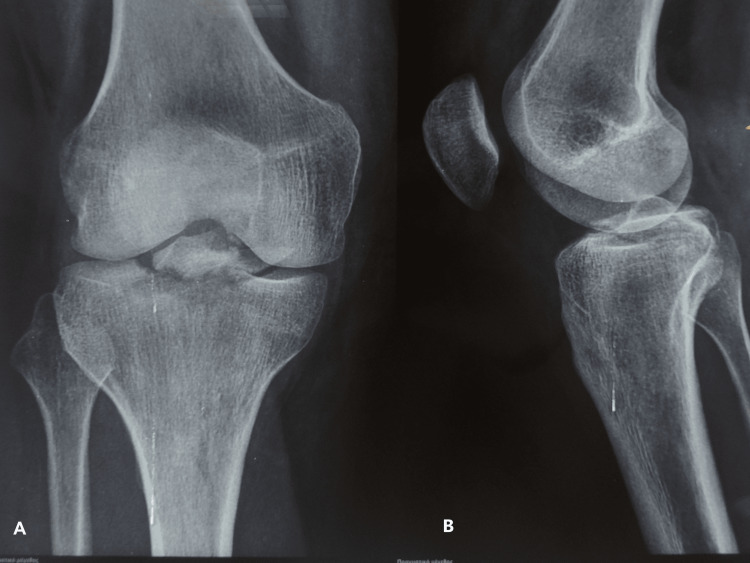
Right knee X-ray. (A) Anteroposterior view. (B) Lateral view. Patient's radiographs depicting the ACL-PCL avulsion fracture. ACL: anterior cruciate ligament; PCL: posterior cruciate ligament
